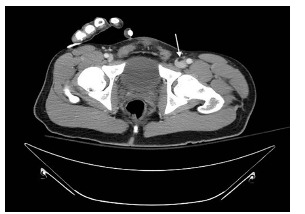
Computed tomography of the pelvis in axial view showing fat fluid level within the left common femoral vein (arrow).
